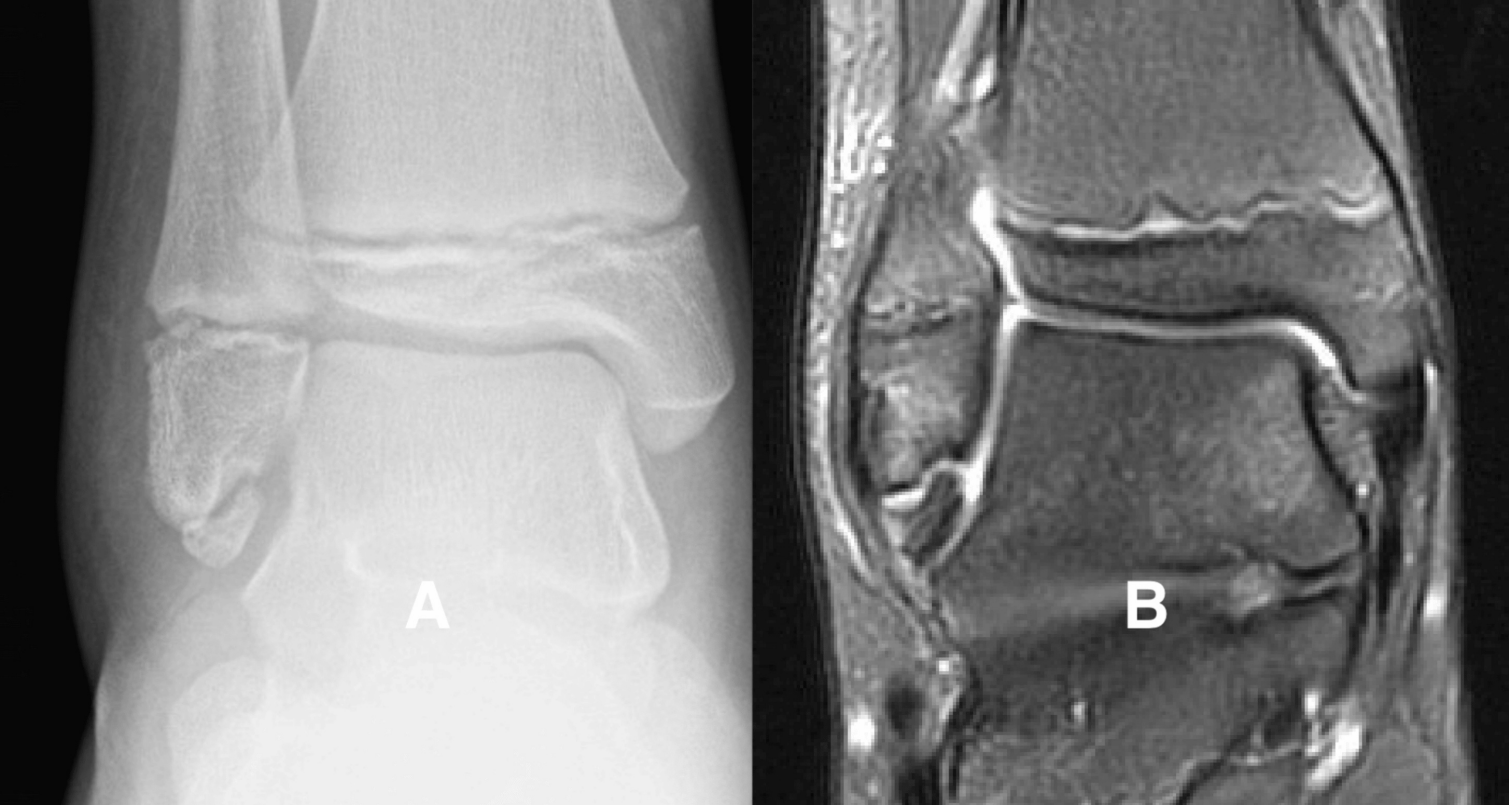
High-intensity fluid sensitive signal between the subfibular ossicle (SO) and the tip of the fibula. Figure 1A is a radiograph showing a well-formed SO present at the tip of the fibula. Figure 1B is a T2-weighted MRI sequence showing a high-intensity signal between the SO and the tip of the fibula.
